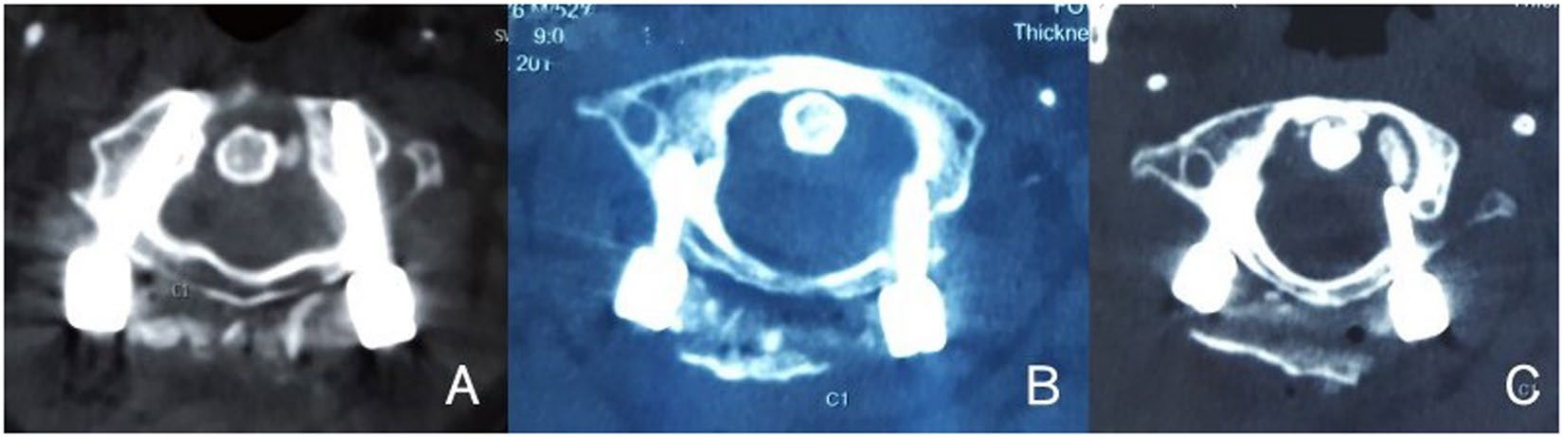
CT images were graded by the correctness of screw placement according to Hojo. (A) Grade 0 (G-0); (B) Grade 1 (G-1); (C) Grade 2 (G-2).
